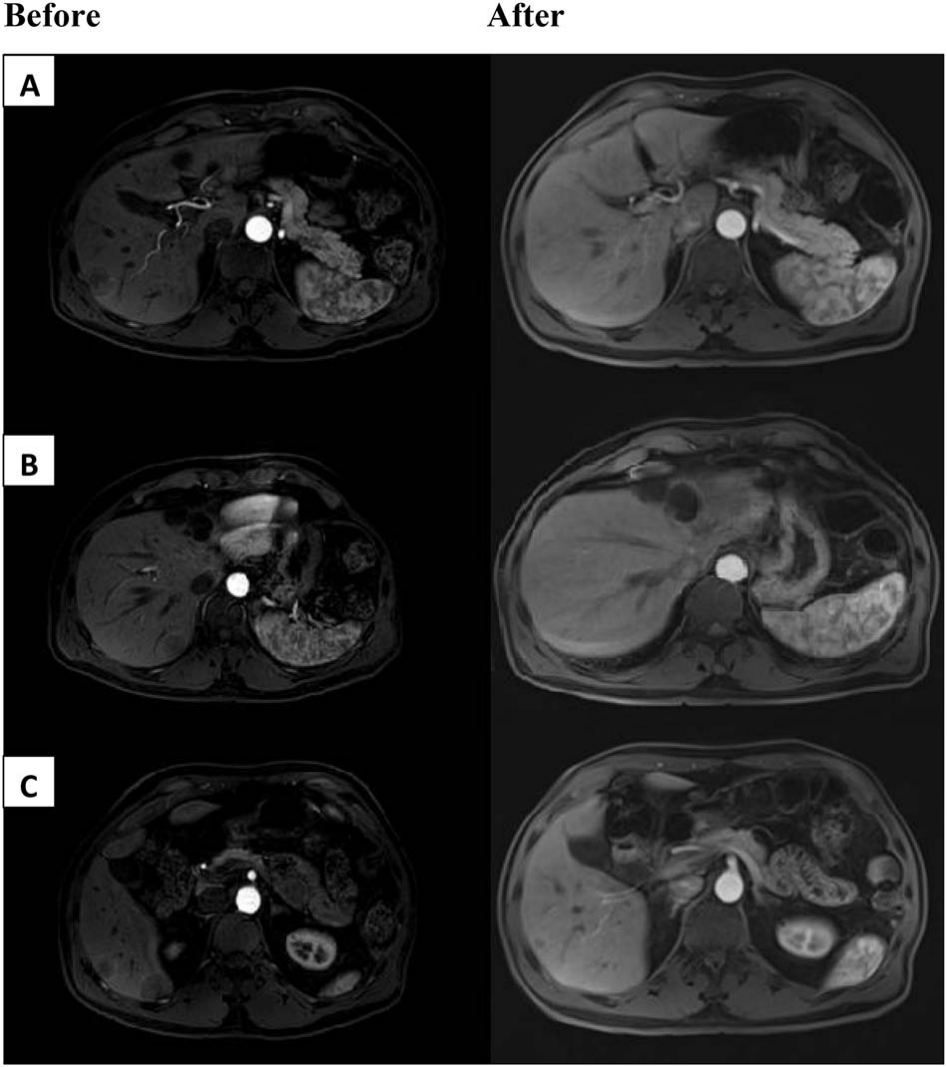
Above are representative images demonstrating severity of patient’s tumor burden, before (left) and after (right) dual anti-HER2 therapy. Patient had bilobar liver metastases ranging from 2.5 to 3 cm in segments 5, 7 and 8. Before images appear different than after due to institutional variation of MRI scanners.
